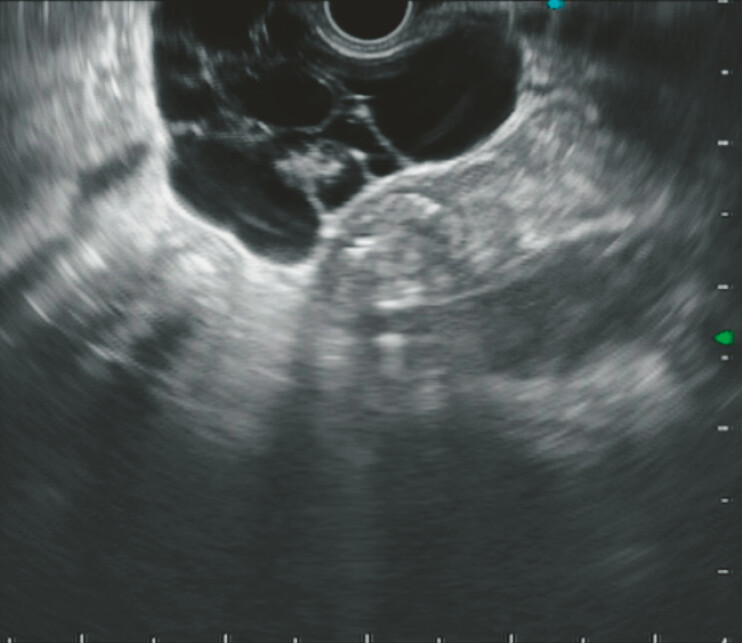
Endoscopic ultrasonography. Hypoechoic lesions, visible space through the stomach lining, and local cystic wall thickening; largest interface measured approximately 5.7 × 4.6 cm.
